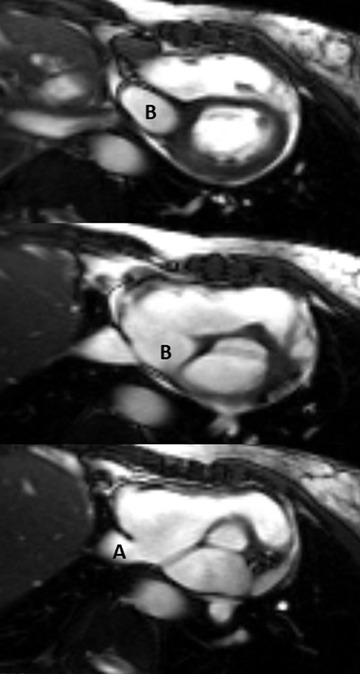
Magnetic resonance images of incidental Abernethy malformation. Short axis steady‐state free precession cine stack demonstrating the abnormal portal vein (B) and the inferior vena cava (A) entering the right atrium
